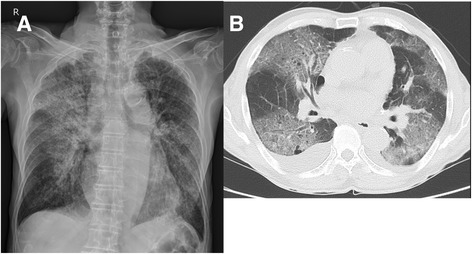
Chest imaging at admission. Chest X-ray showing diffuse haziness which is dominant in right lung (a). Chest computed tomographic scan showing ground glass opacities in both lungs with pleural effusion dominant at the right hemithorax (b)
